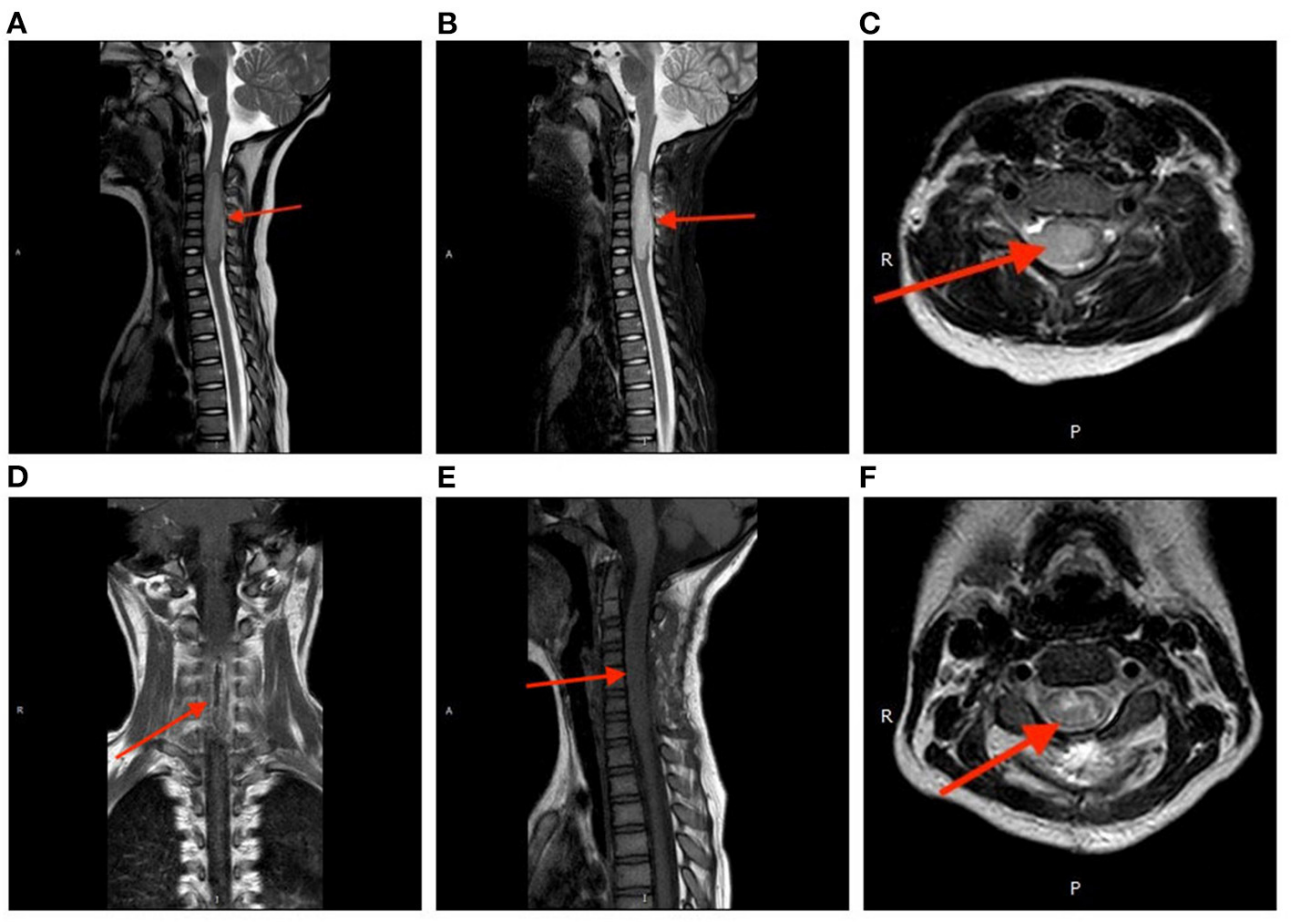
Perioperative magnetic resonance imaging. The tumor showed isointensity on T1-weighted imaging (A) and slightly hyperintensity on T2-weighted imaging (B). After administration of contrast medium, the lesion was heterogeneously enhanced (C). Postoperative spinal MRI confirmed a subtotal resection of the tumor (D) coronal, (E) sagittal, (F) axial.
